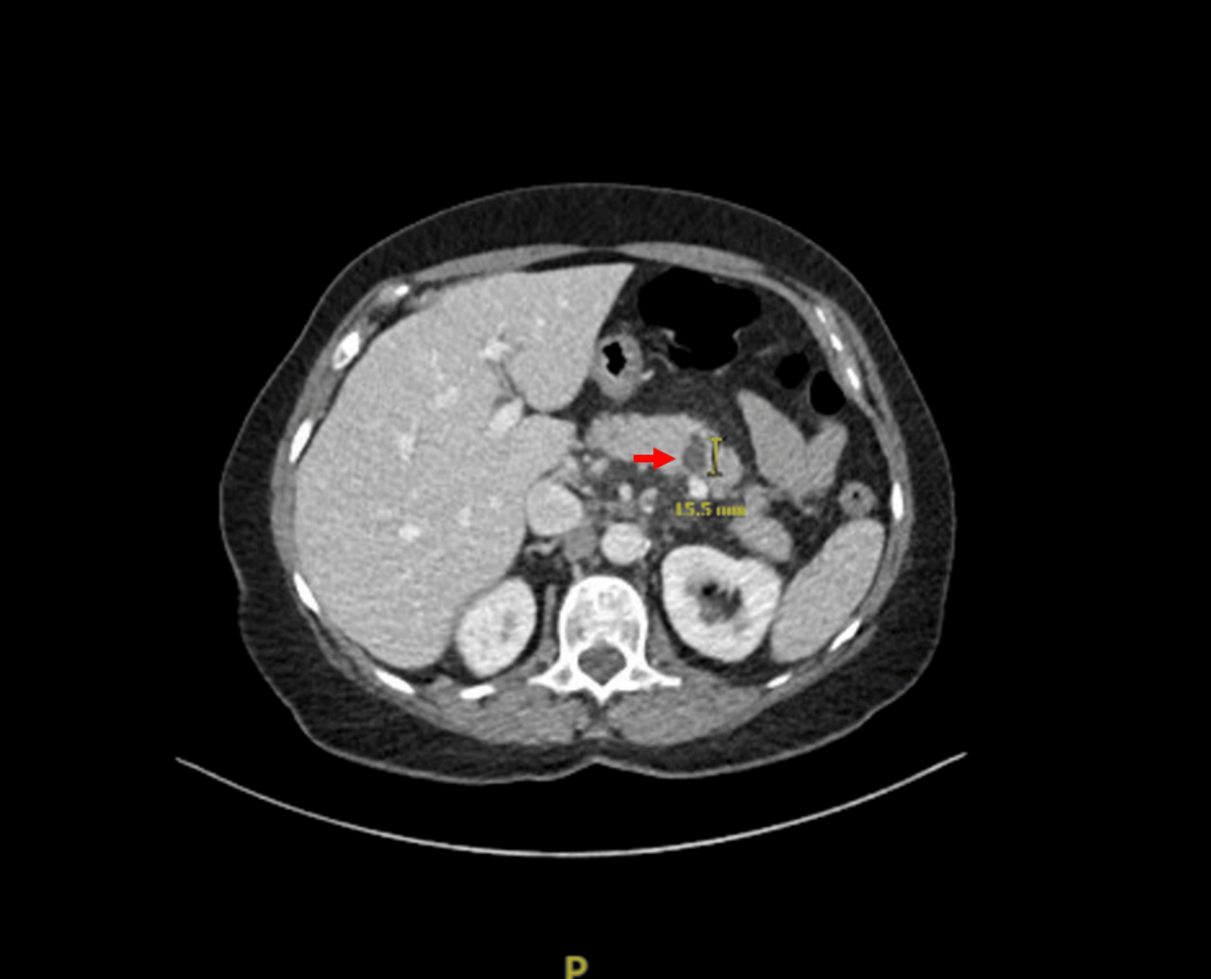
CT scan of abdomen showing 1.6 cm cystic lesion (red arrow) in the mid-pancreatic body.
